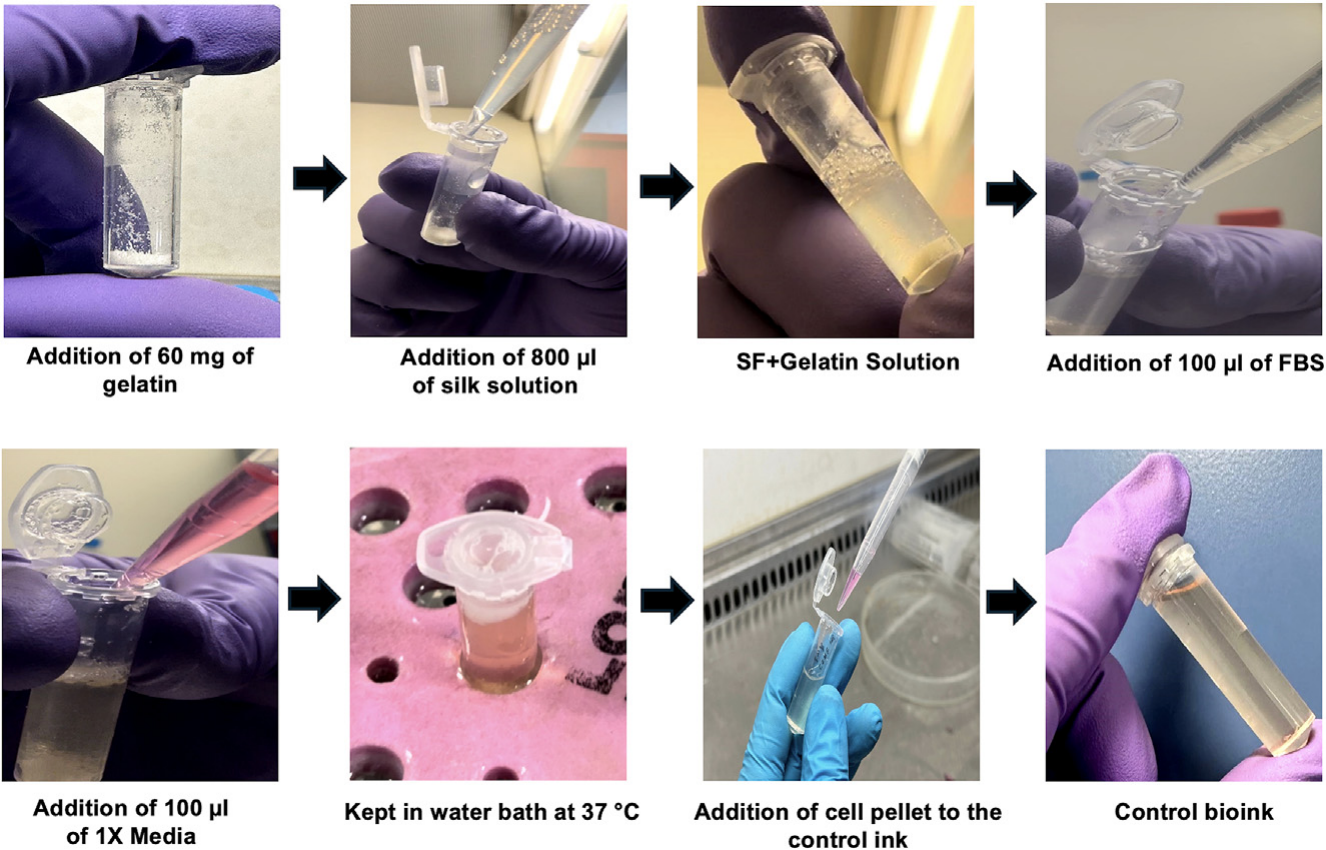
Steps involved in the preparation of the control bioink (SF-G)15.Preparation of the magnetic bioink (SF-G-MNP) (Figure 3).a.Cleanse the MNPs with deionized water 2–3 times. These are morphologically elongated, having an aspect ratio of 3.3 ± 0.5, with an average length of 178 ± 16 nm and a width of 55 ± 7 nm.b.Dry them in an oven at 100°C until completely devoid of water.c.Sterilize the MNPs for 1 h inside the laminar flow hood with a 90 mm petridish while exposed to UV light.d.Ultrasonicate 5 mg of the MNP in 500 μL of PBS for 5 min at 50% amplitude, frequency 20 kHz.e.Add the MNP suspension to the control acellular ink.f.Adjust the volume to form 5 mg/mL of the MNP containing acellular bioink.g.Suspend 1 × 10^7^ cells/mL to the acellular MNP containing acellular bioink to form the SF-G-MNP bioink.
